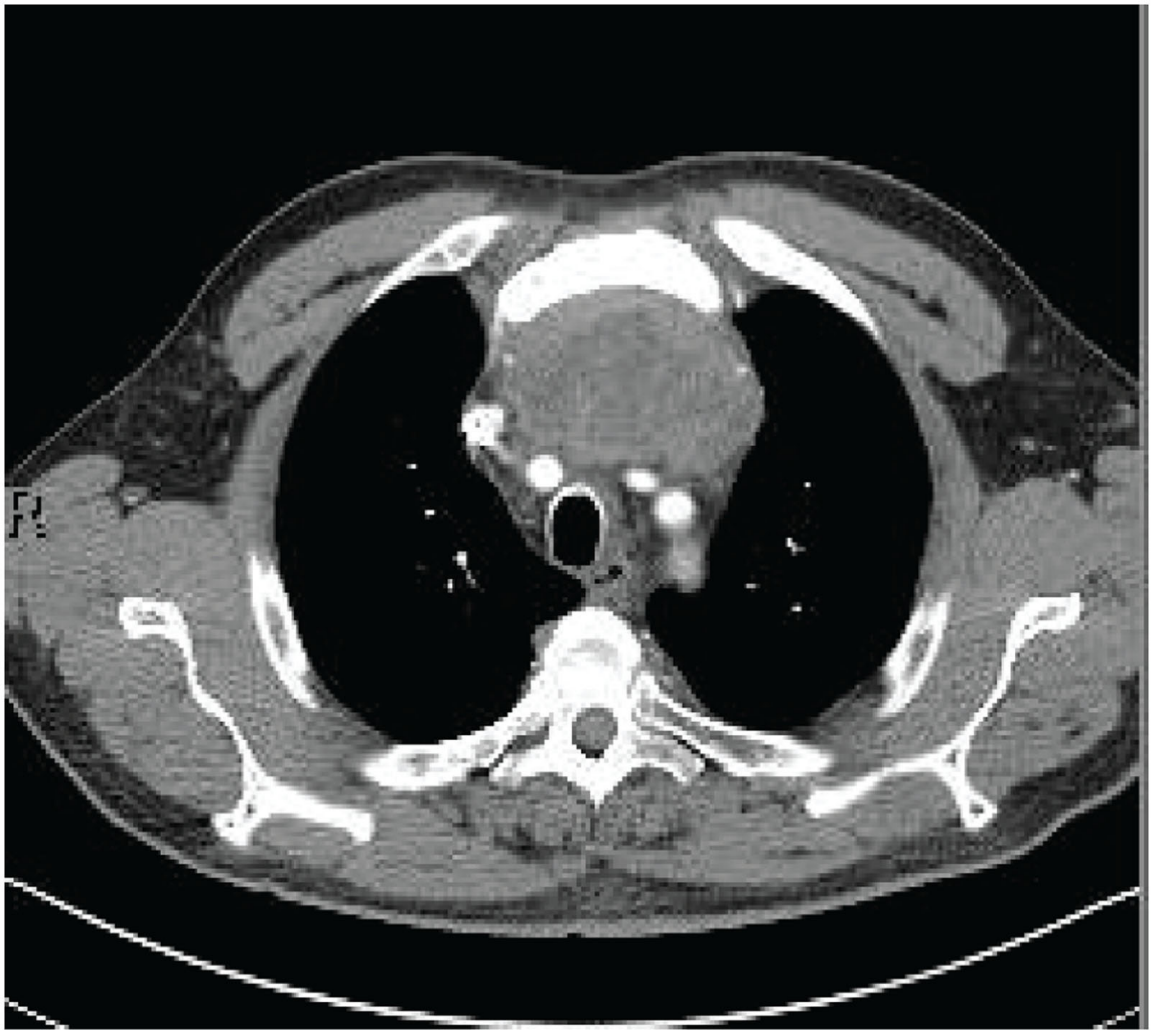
CT scan prior to radiation.
